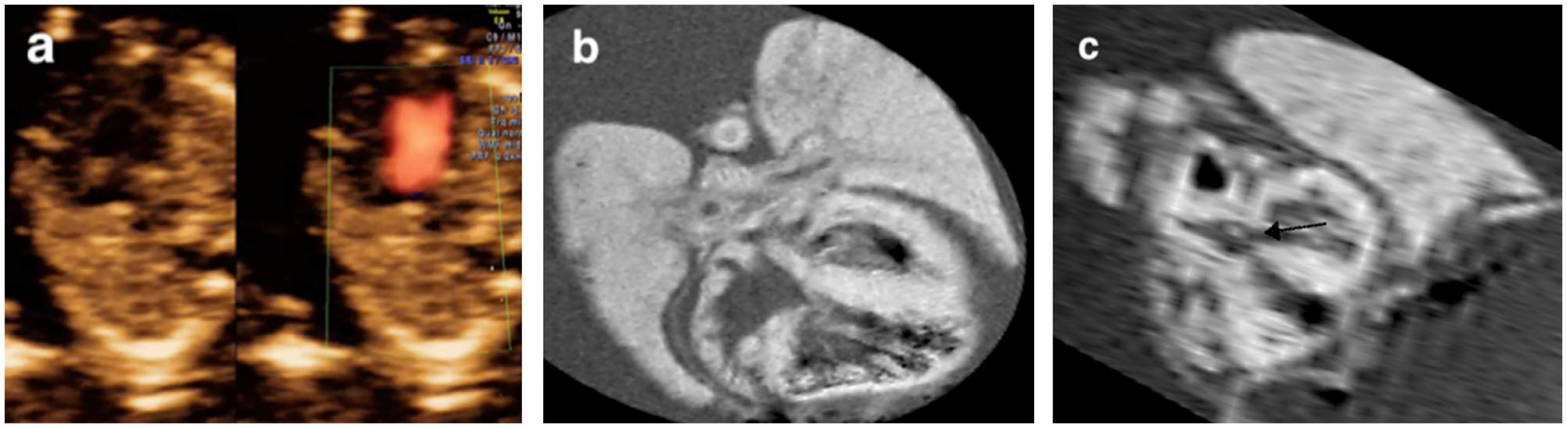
Discordant findings of postmortem MRI compared with prenatal ultrasound. (a) A fetus presented with an AVSD on the four-chamber view and was terminated at 13+0 gestational weeks (Case 1 in Table 2). Postmortem MRI showed intact atrioventricular septal (b) and left ventricle outflow tract showed malalignment ventricular septal defect (c, black arrow).
